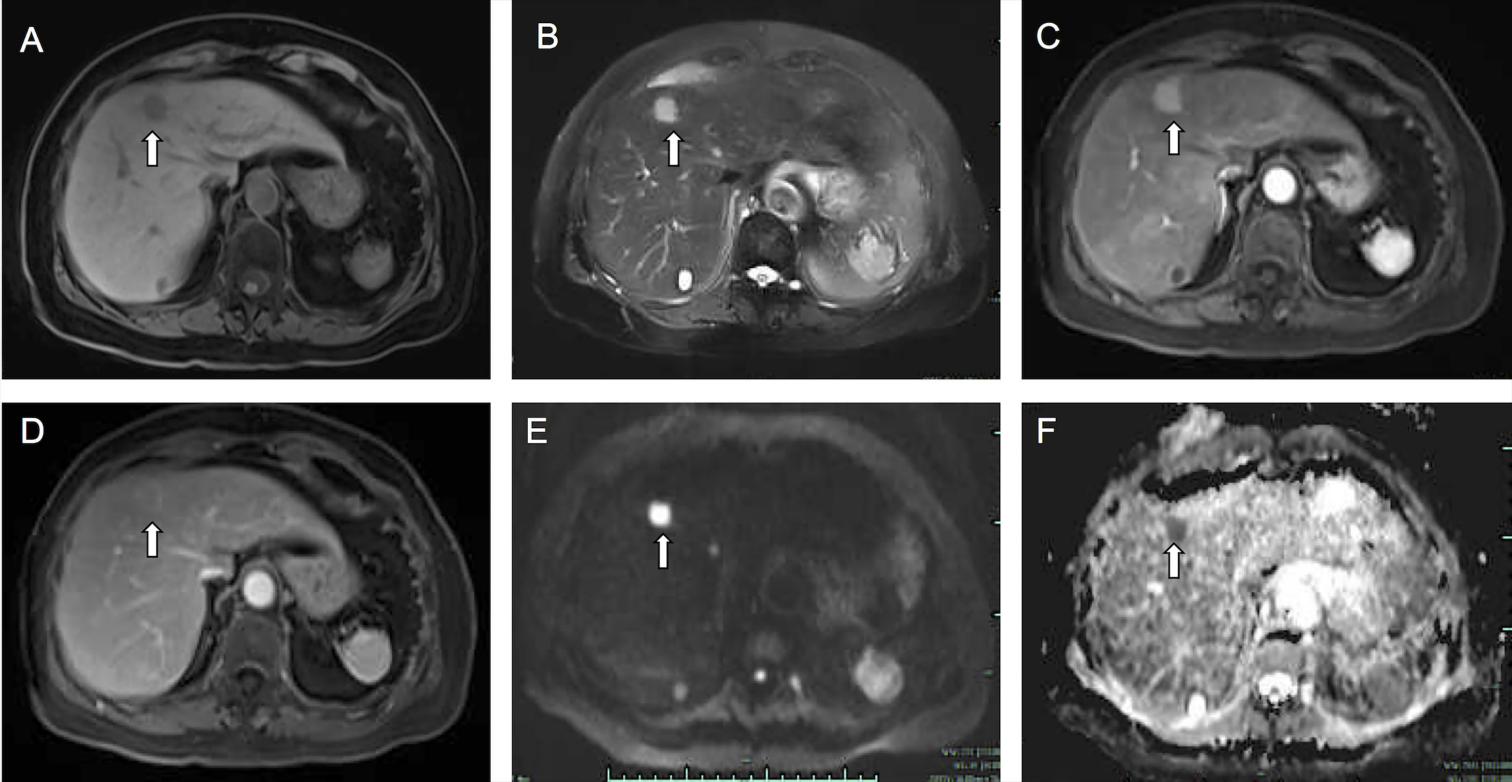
Fat-saturated T1-weighted image (A) showed a hypointense lesion measuring 17 mm in the segment 4 of the liver. The lesion showed hyperintensity on T2-weighted image (B). On enhanced T1-weighted images, the lesion showed obvious enhancement in arterial phase (C), and in portal phase (D) the lesion showed slight washout and perinodular enhancement. On DWI (E) and ADC (F) images, the lesion showed a significant diffusion restriction. The white arrow points to the lesion.
